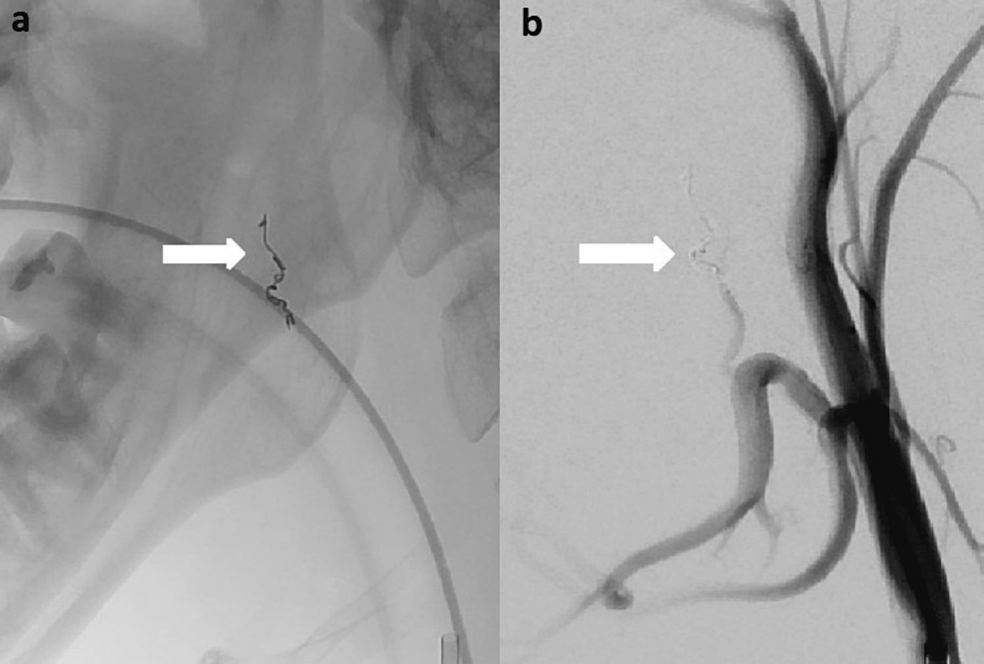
a) X-ray of the face (lateral view) showing microcoils in the ascending palatine artery. b) Post-embolization digital subtraction angiography (lateral view) of the ECA showing occlusion of the ascending palatine artery ECA: External carotid artery
